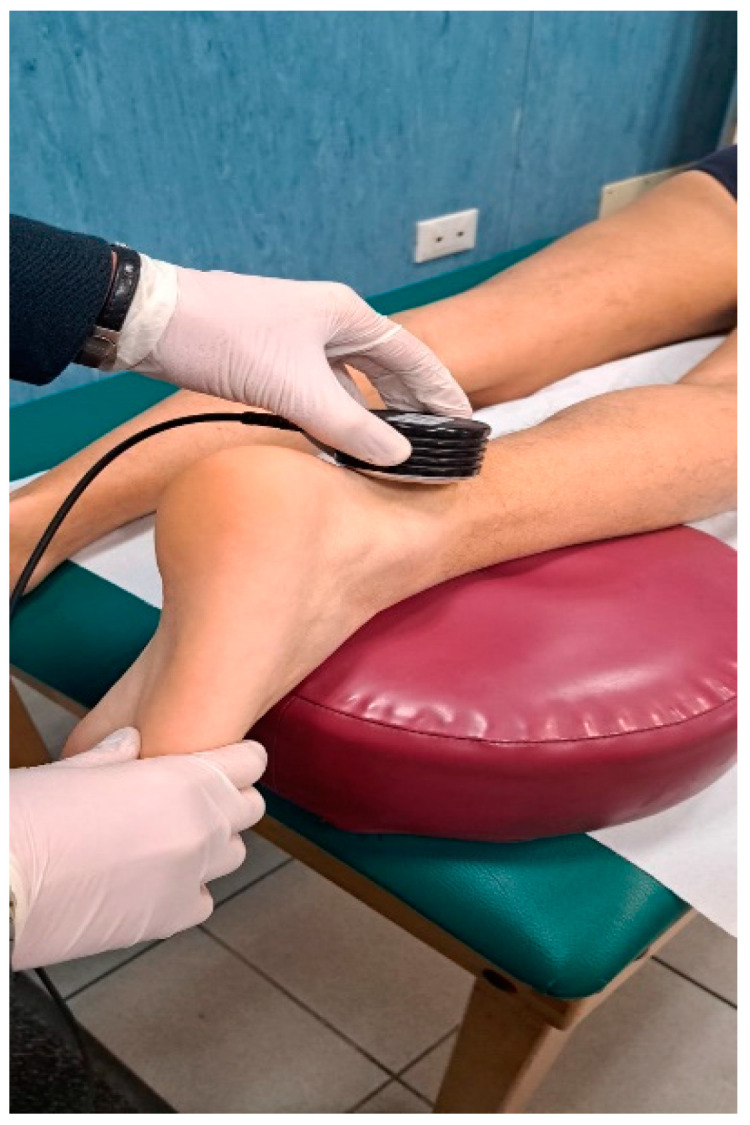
Ultrasound therapy for mid-portion AT.
